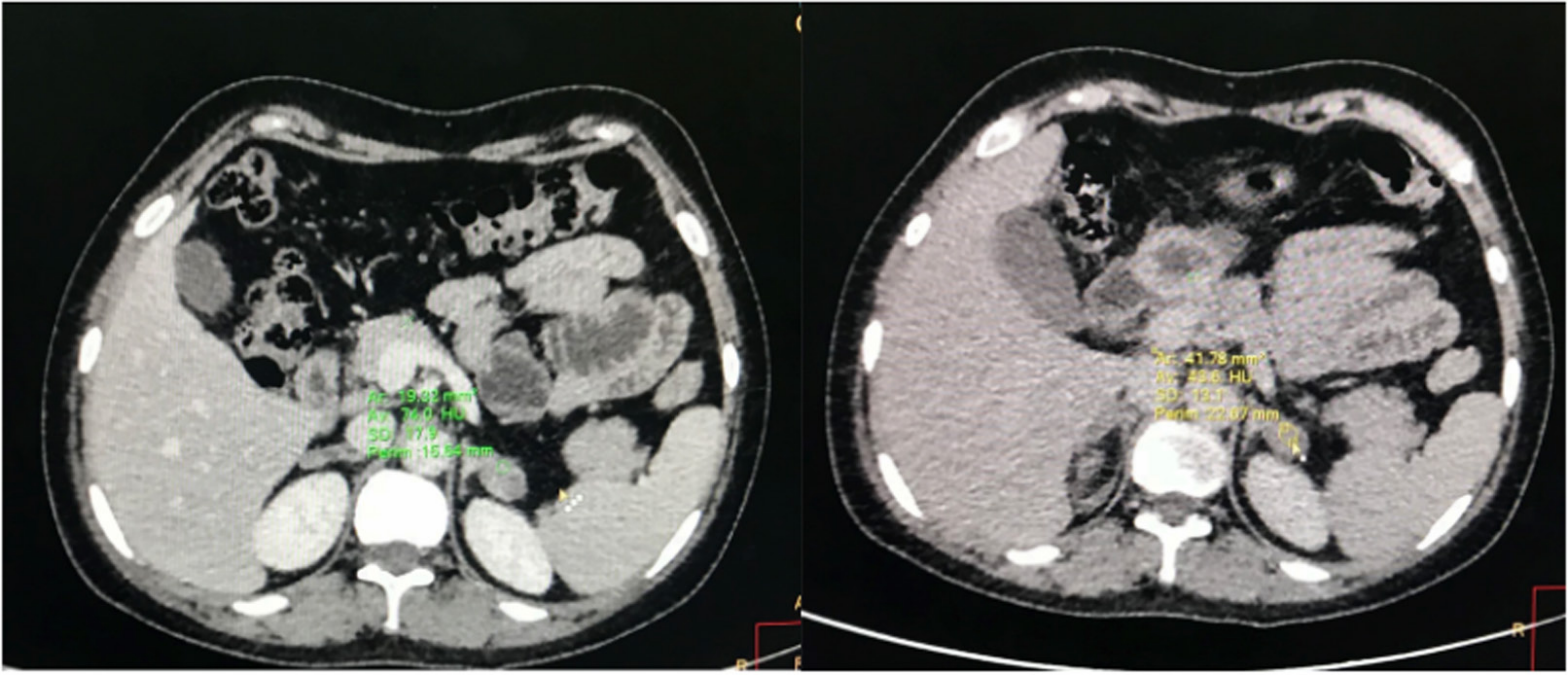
Axial CT image showing bulky left adrenal with a well‐defined homogenous nodular lesion in the lateral limb. On portal venous phase, the lesion shows homogenous enhancement (HU+74), and on delayed phase, it shows washout of contrast (HU+43) with respect to the portal venous phase
